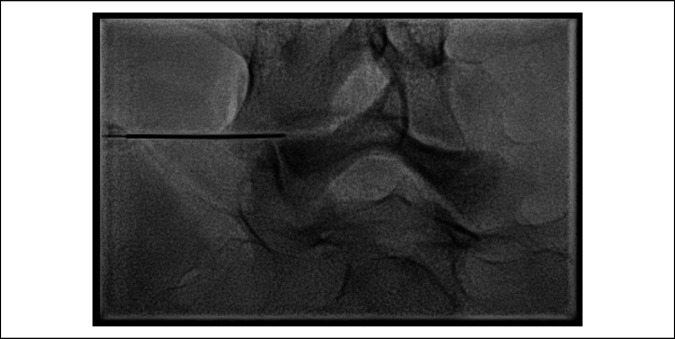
Radiograph showing fluoroscopic-guided injection performed by interventional radiology at L5 on the left while the patient is lying prone on her left to potentially relieve pain caused by the left L5 pars defect.^17^
